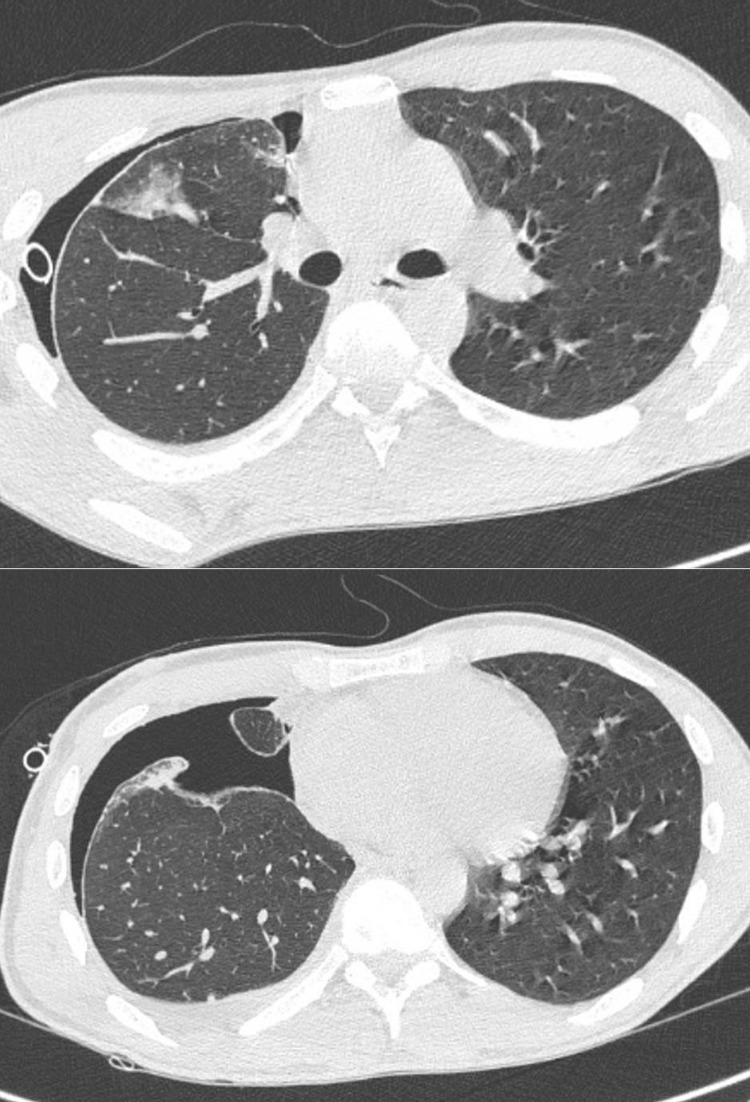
Chest computed tomography performed 10 days following chest tube insertion showing non-expandable right lung
